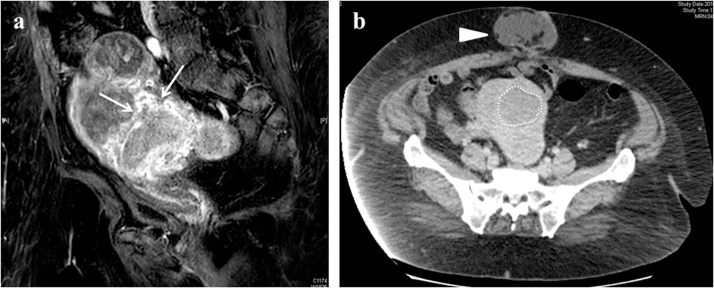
(a) Pelvic MRI (sagittal section of dynamic MRI) disruption of subendometrial enhancement in from uterine anterior wall to the fundus (white arrow). (b) Contrast-enhanced CT (horizontal section) tumor lesion in uteri (white broken line) and umbilical hernia (white triangle).
